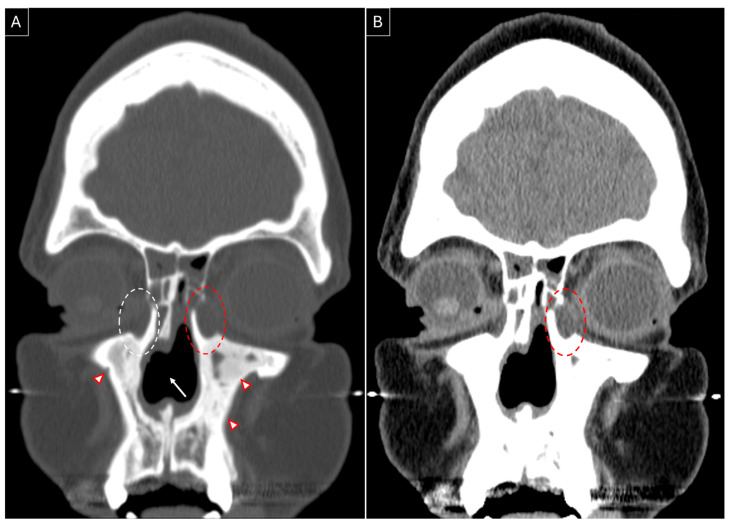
Non-contrasted sinus CT, coronal section, showing left-sided dacryocystocele (red dotted circle) in contrast to the right-sided lacrimal sac (white dotted circle). Presence of a nasal septal perforation (white arrow) and osteitic bone remodelling (red arrowheads) can be noted in both bone (A) and soft tissue (B) windows.
